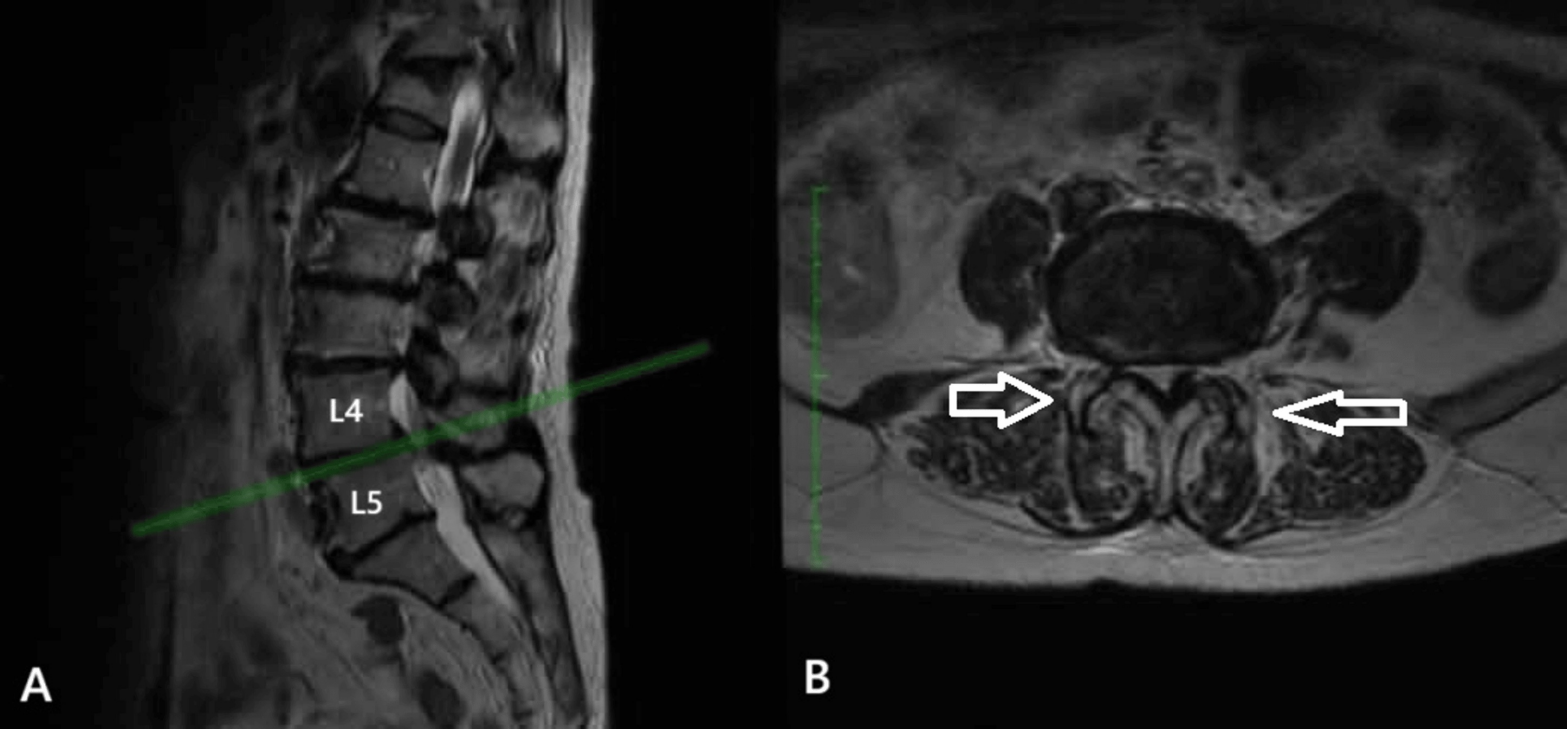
(A) T2-sagittal view and (B) T2-axial MRI views of the lumbar spine in third case. At the L4-L5 level, the arrows with the white outline indicate the bilateral hypertrophic facet joints (grade 4 according to Grogan), with accompanying left facet subluxation.
